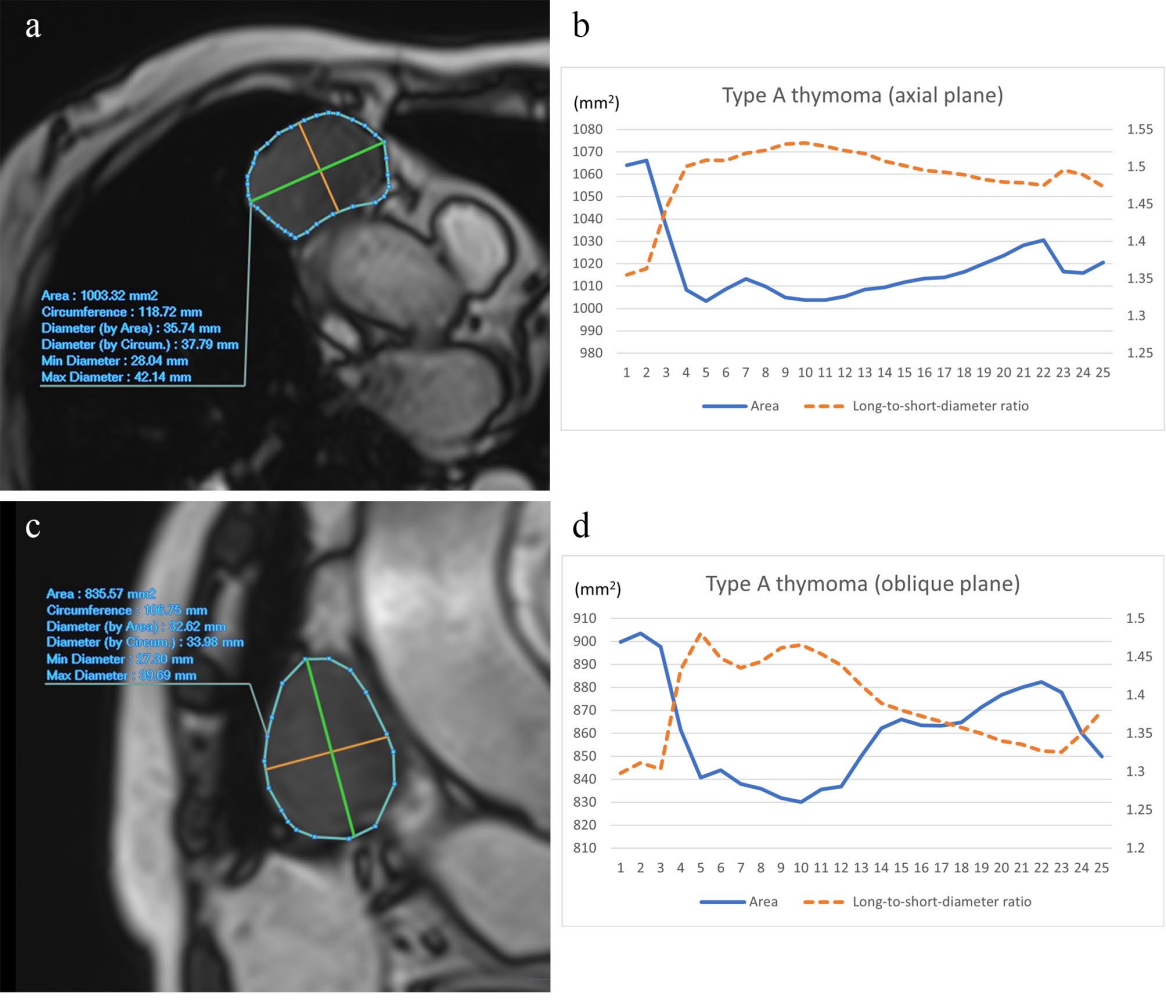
A 64-year-old woman with low-risk thymoma (type A). Axial (a) and oblique (c) cine MR images of low-risk thymoma (type A) in the right anterior mediastinum, accompanied by graphs representing the time course of area and long-to-short diameter ratio in axial (b) and oblique planes (d). In the axial plane, ∆long-to-short diameter ratio and ∆area were 10.58% and 5.89%, respectively. In the oblique plane, ∆long-to-short diameter ratio and ∆area were 10.50% and 8.14%, respectively
